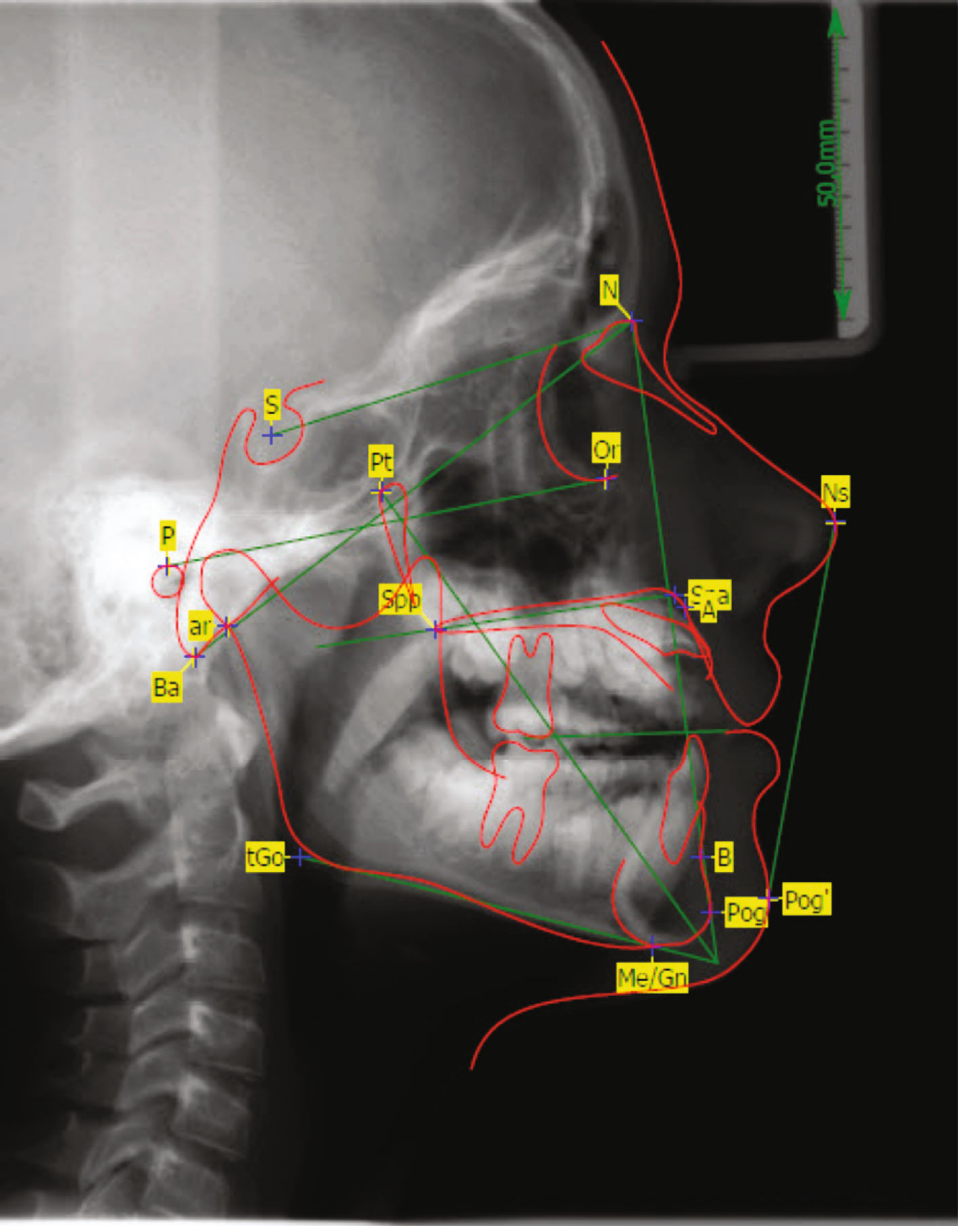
Lateral cephalometric radiographs at the age of eleven; for abbreviations, see also Table 1.
